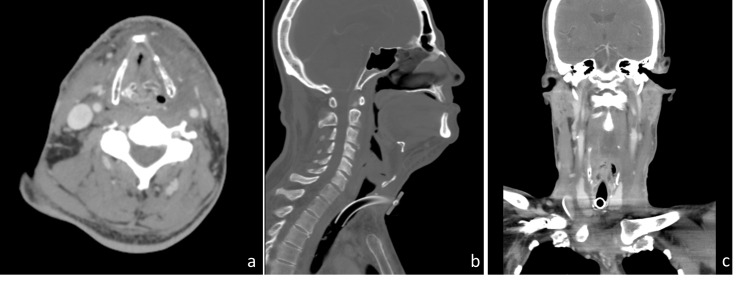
One Month Follow-up CT Scan Follow-up (a) axial, (b) saggital, and (c) coronal CT scan one month after the completion of radiation. Diffuse mucosal edema and enhancement in the pharyngeal mucosal space, oral cavity, and larynx are related to postradiation changes. Previously seen large hypodense mass centered in the left neck has significantly decreased in size.
